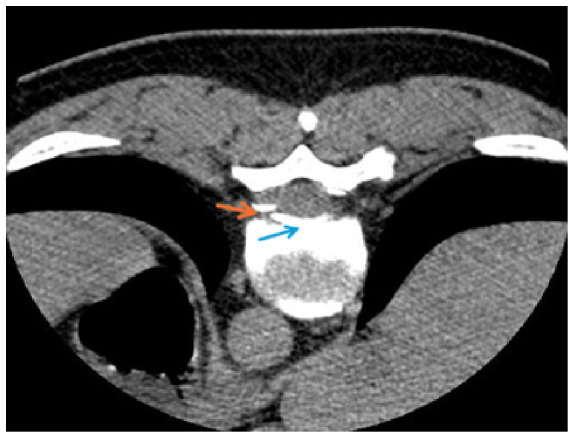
CT myelography image obtained after intrathecal administration of iodinated contrast material (blue arrow indicates intrathecal contrast). At the T8 level, there is focal contrast extravasation through a dural defect (orange arrow), with leakage into the ventral epidural space between the dural layers, confirming the site of the cerebrospinal fluid (CSF) leak.
